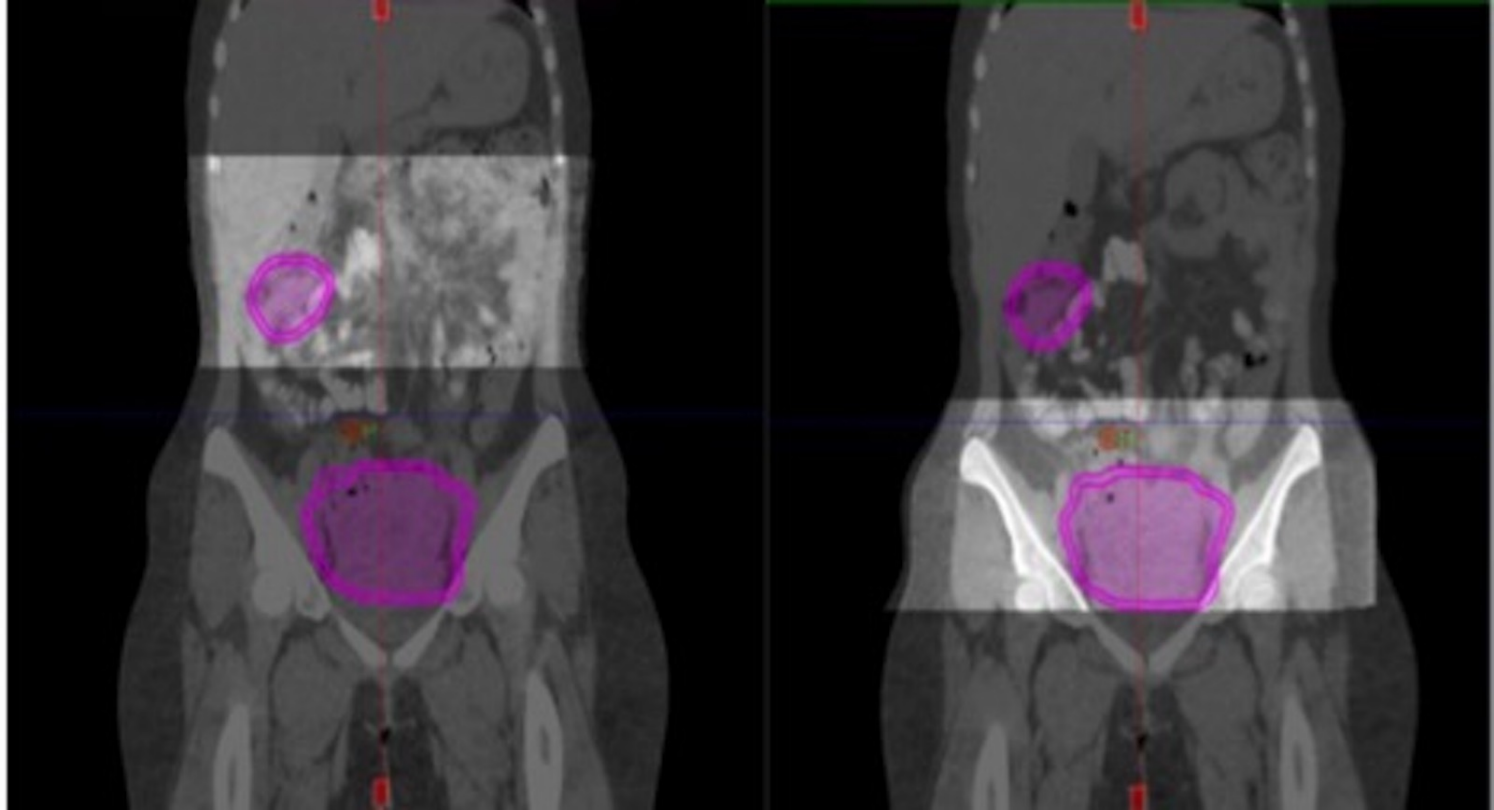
Superior and Inferior abdominal Cone-Beam CT during radiation treatment.
